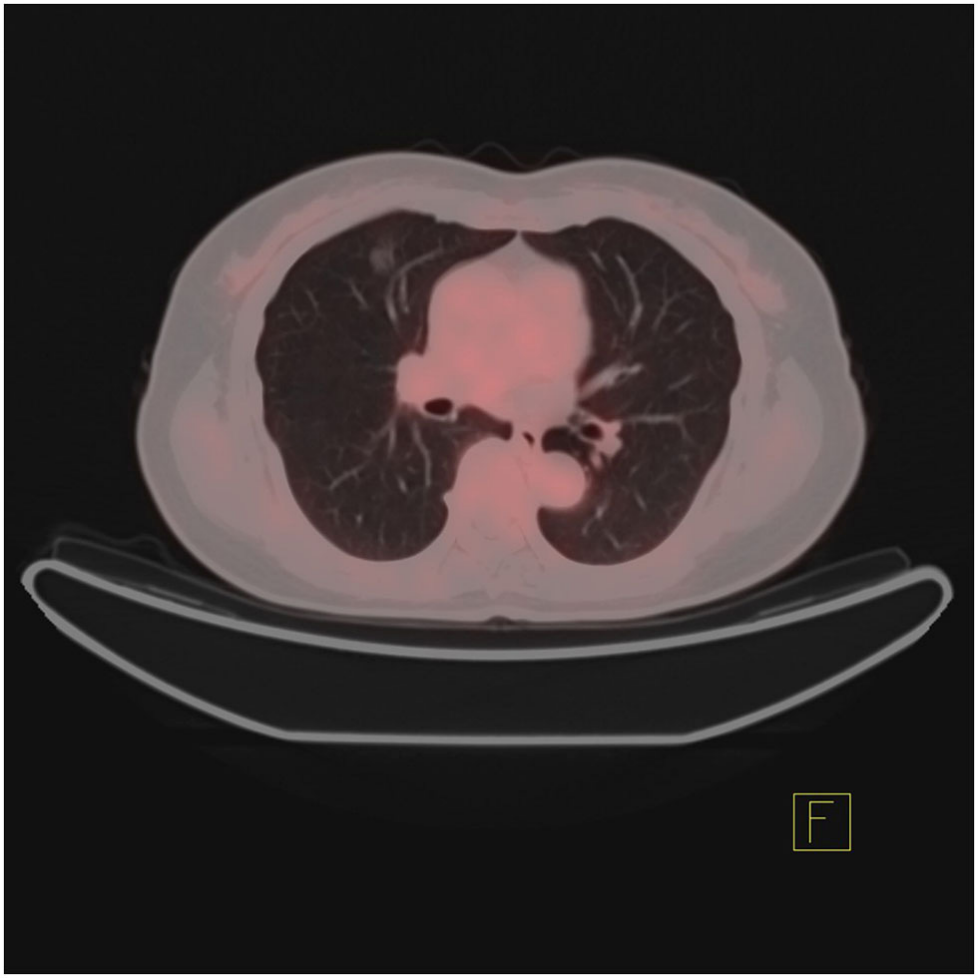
Non-metabolic invasive adenocarcinoma on PET/CT.
